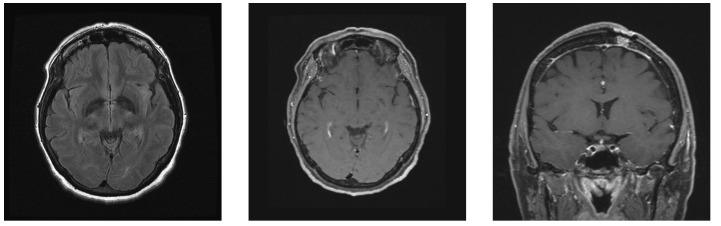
Magnetic resonance imaging demonstrating further lesion resolution with a return to near-normal regional anatomy.
